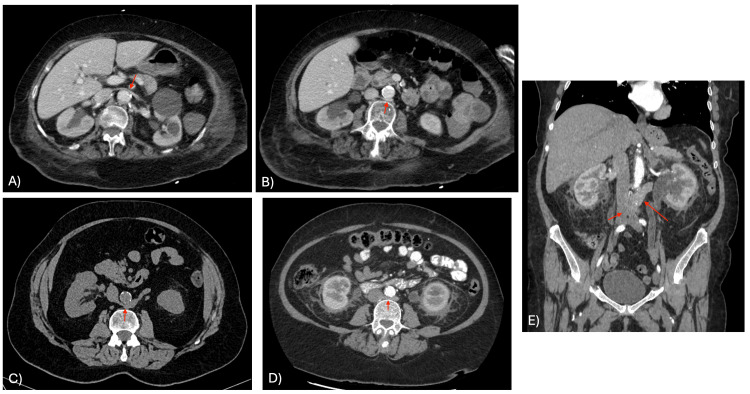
Type I, II, and III RLRV (A) Case 3: Contrast-enhanced CT demonstrating a circumaortic (type III) RLRV; the arrow points to the superior preaortic renal vein. (B) Case 3: Contrast-enhanced CT demonstrating the same type III RLRV, with the arrow indicating the retroaortic left renal vein. (C) Case 1: Axial non-contrast CT showing a type I RLRV (arrow) coursing posterior to the aorta and draining into the IVC at the L1-L2 level. (D) Case 2: Type II RLRV (arrow) draining into the IVC at L4. (E) Case 2: Coronal CT view demonstrating the type II RLRV; the short arrow indicates the entry point of the left renal vein into the IVC at L4, while the long arrow shows its retroaortic course. RLRV: retroaortic left renal veins, CT: computed tomography, IVC: inferior vena cava
